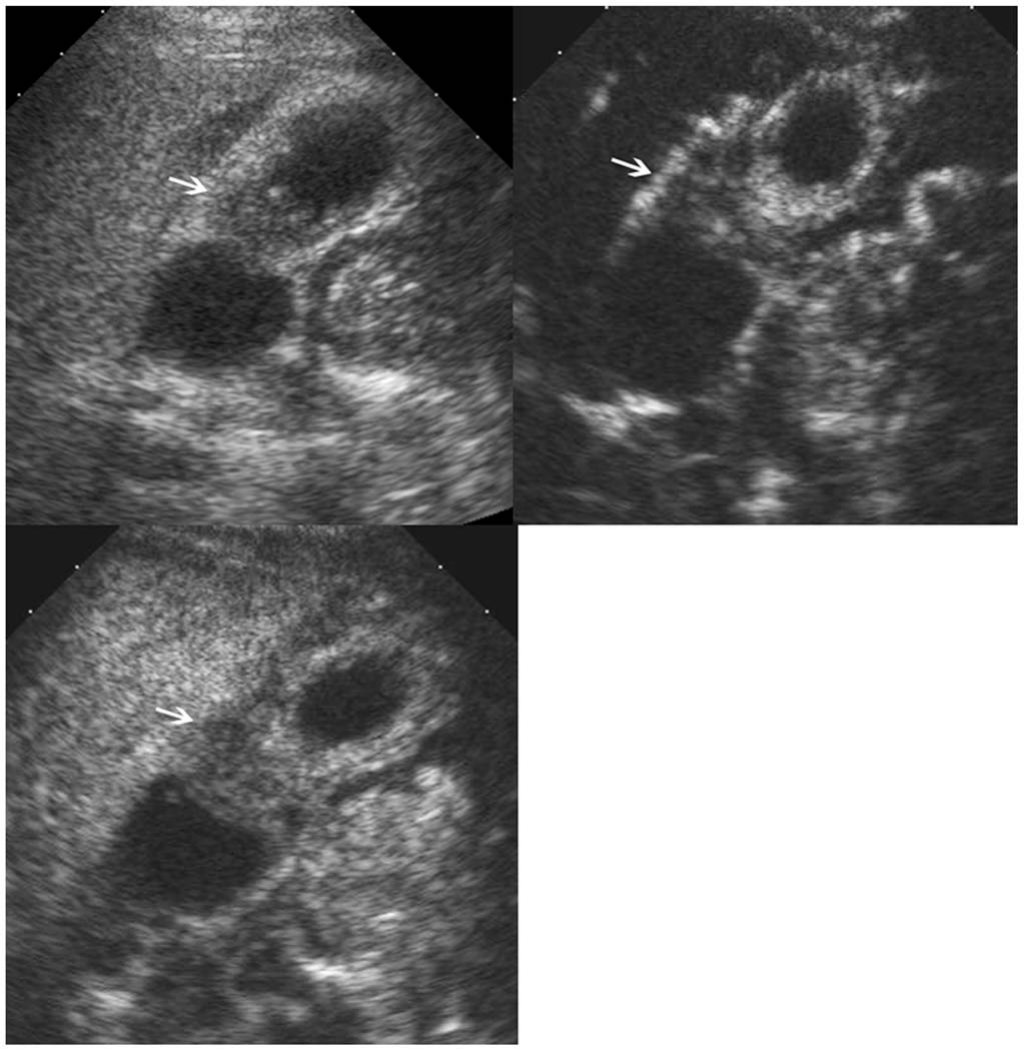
Adenomyomatosis in gallbladder. Upper left, conventional ultrasound shows a slight hypoechoic mass (arrow) in the gallbladder; Upper right, the lesion (arrow) shows inhomogeneous hyper-enhancement during the arterial phase; Lower left, the lesion (arrow) shows hypo-enhancement during the venous phase.
